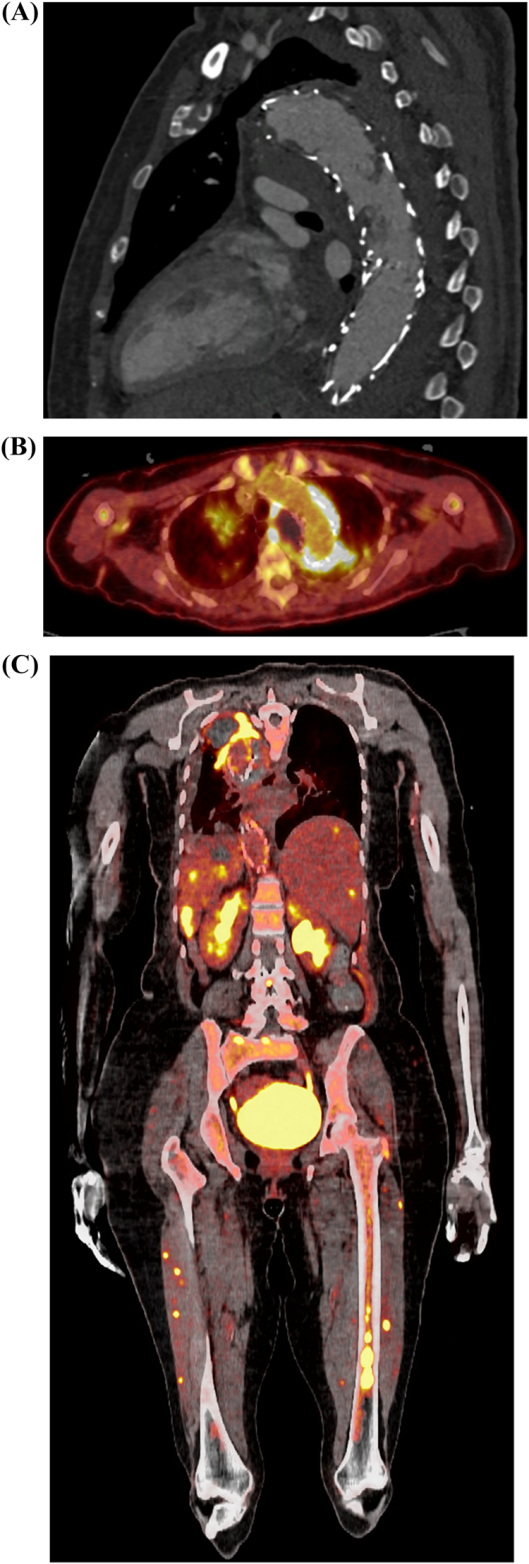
Cross‐sectional imaging of aortic sarcoma after prior thoracic endovascular aneurysm repair. (A) CTA sagittal view demonstrating luminal thrombus within endograft. (B) PET‐CT axial view demonstrating hypermetabolic activity around endograft. (C) PET‐CT coronal view demonstrating hypermetabolic activity of thoracic aorta, spleen, kidney, liver, and lower extremity osseous lesions.
